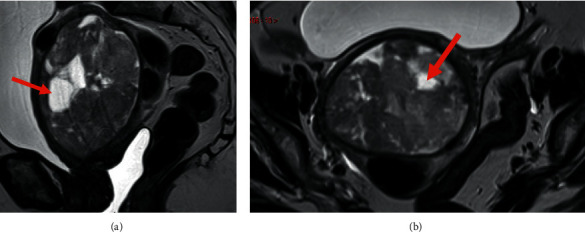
Pelvic MRI of MMMT: (a, b) sagittal (a) and axial (b) T2WI showing a large intrauterine tumor with mixed heterogeneous signal, cystic component showing high signal intensity (arrow).
